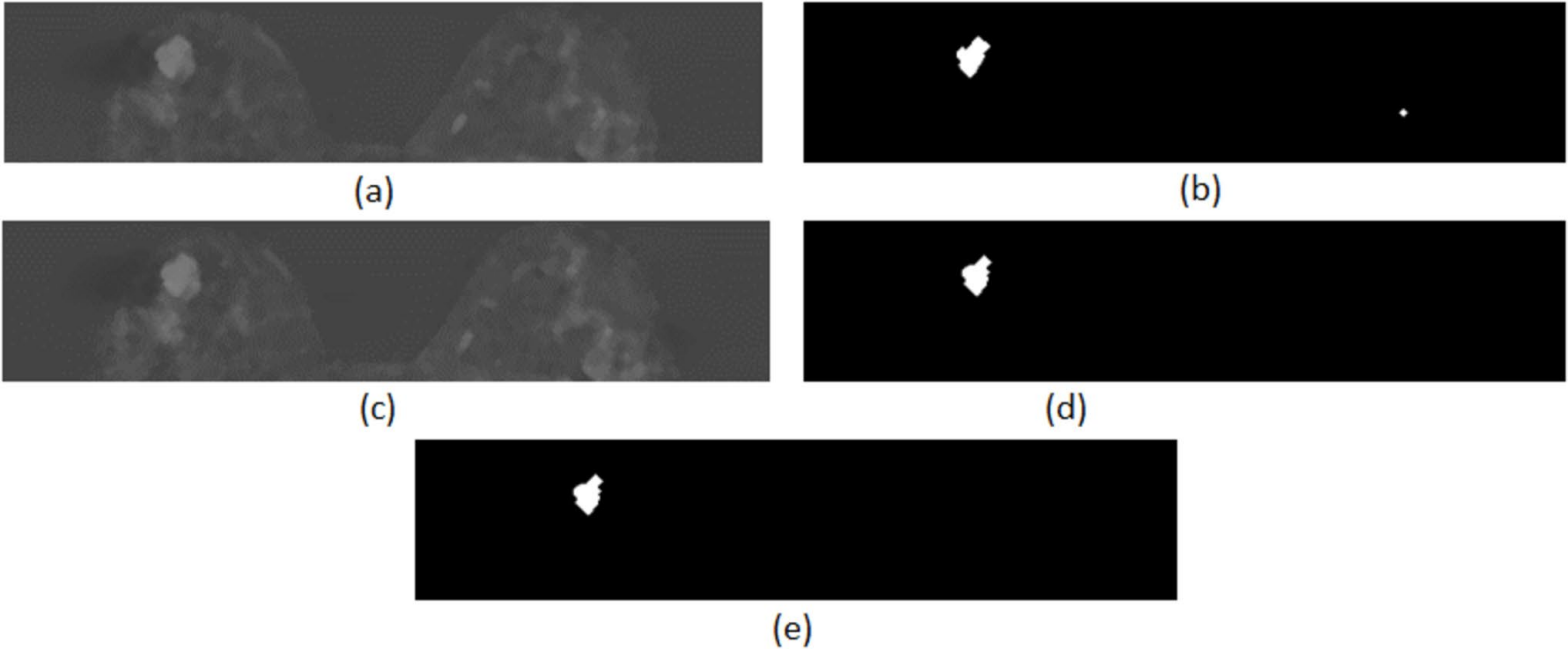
Initial ROI extraction in an MRI slice: a image *I*_*flt*2_, b binary image *I*_*otsu*2_, c image *I*_*flt*3_, d binary image *I*_*otsu*3_, and e initial ROI extraction for the MRI slice by intersection of the two generated binary images—image *I*_*otsu*_
